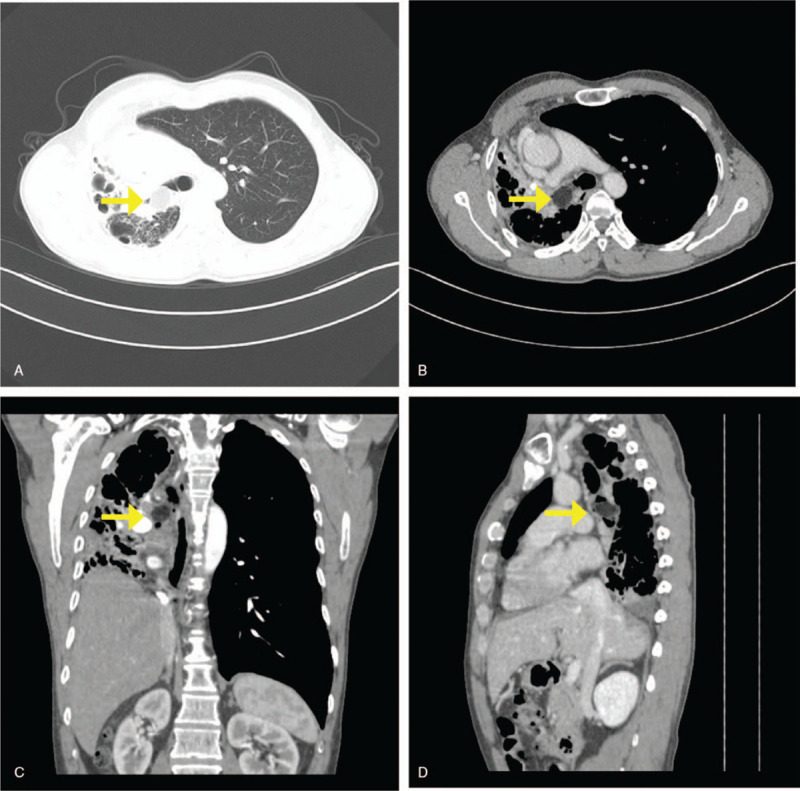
Chest CT showing an extra-bronchial extension of tumor with low attenuation suggesting a fat-containing lesion (arrow). There is an obstruction of the right bronchus intermedius causing complete atelectasis of the right lung with encysted pleurisy and mediastinal shift toward the right; multiple cystic cavities, bronchiectasis, and obstructive pneumonia complicated by pleural effusion could be seen in the right lung.
